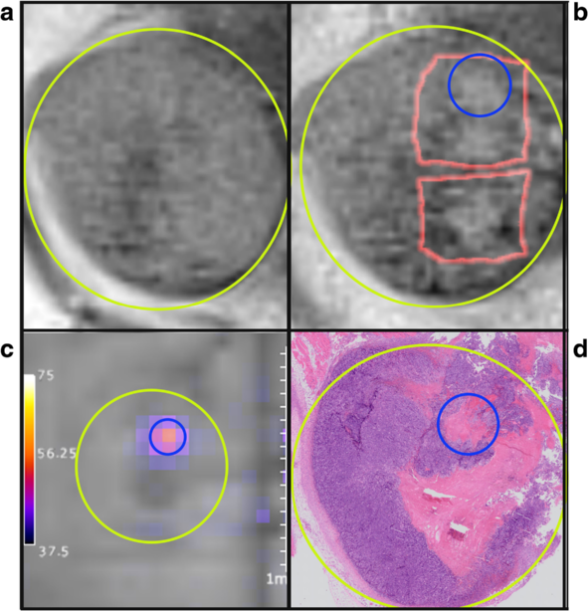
Comparison of the tumor on magnetic resonance (MR) imaging and pathology. T2 weighted (T2W) MR images before (a) and immediately after (b) 3 ablation spots. *Yellow circle* is the tumor, *red squares* are the thermal dose overlay. c:Temperature map (echo planar imaging (EPI) sequence) of one focal spot corresponding with *blue circle* at pathology and T2W image after treatment. d hematoxilin and eosin slice of the tumor 3 days after treatment. Showing a focal necrotic region and a confluent necrotic region, caused by heat distribution resulting in fusion of the lesions in the days after treatment
